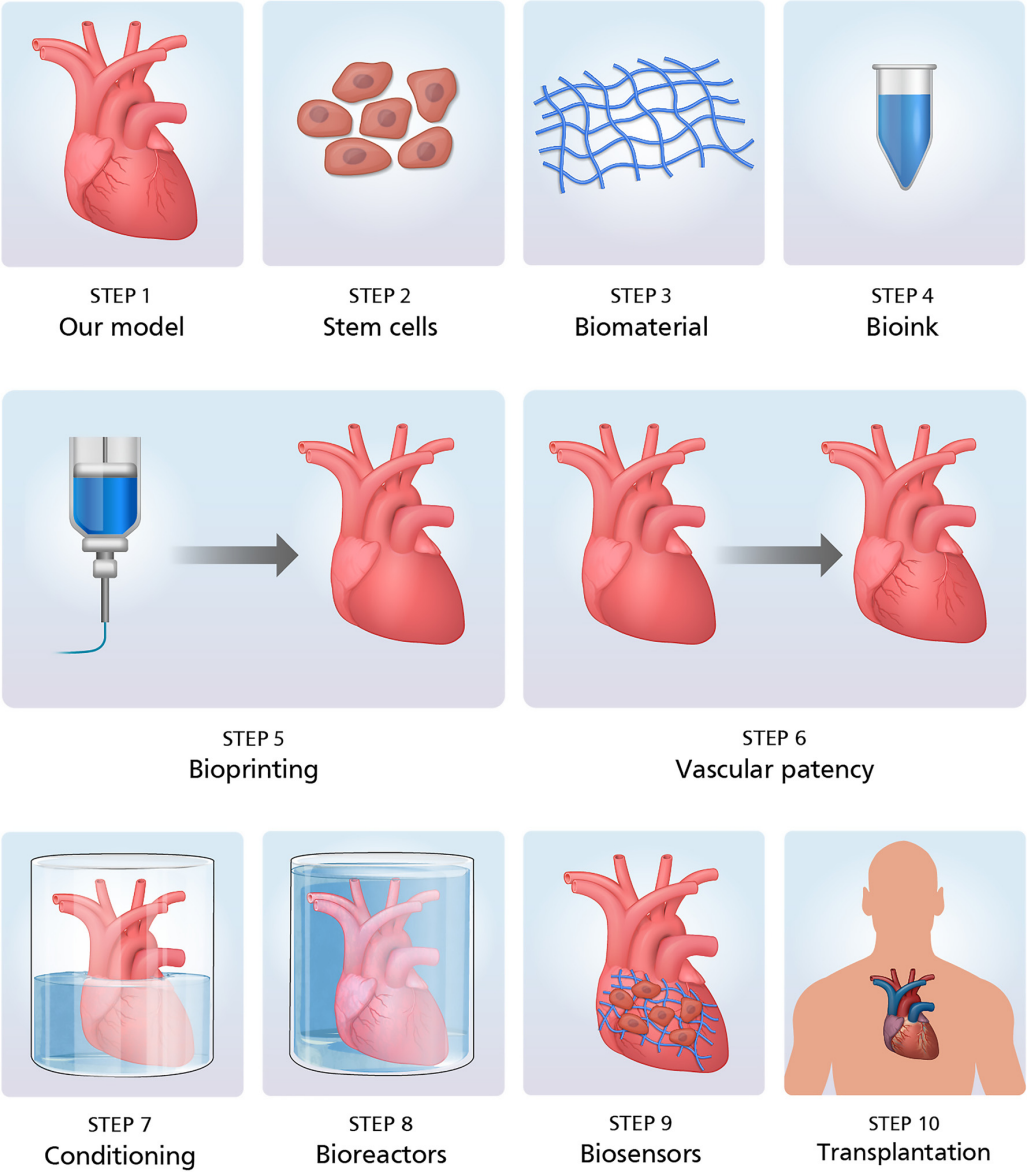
Process for bioprinting human hearts—patient MRI images are used to model the heart.
